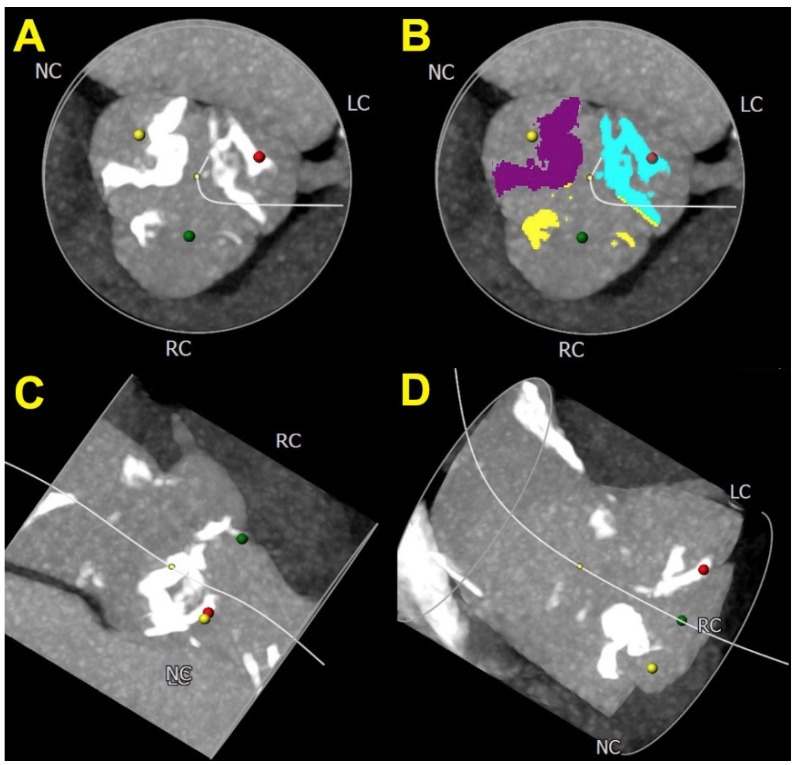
Computed tomography preparation for TAVI depicting very calcified aortic valve in different projections. LC—left coronary cusp, NC—non-coronary cusp, RC—right coronary cusp, (A) calcifications on each cusp of the aortic valve, (B) calcification depicted in different colors to facilitate the calculation of aortic valve calcification score, (C) aortic valve at the particular position provides better visualization for TAVI (RAO 99°, caudal 51°), (D) aortic valve at the particular position to provide better visualization for TAVI (RAO 7°, caudal 1°).
